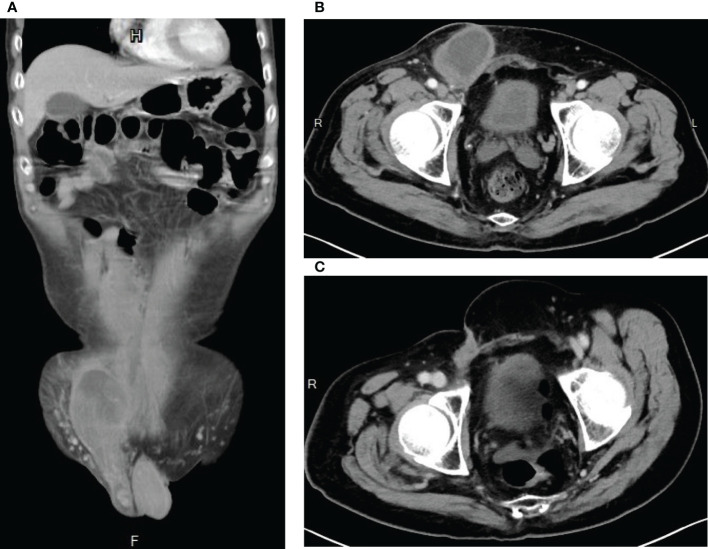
Computed Tomography images showing evidence of tumor shrinkage (A) after neoadjuvant radiotherapy plus conversion surgery, (B) after adjuvant chemotherapy AIM (ifosfamide, Mesna, and doxorubicin) and MAID (Mesna, doxorubincin, ifosfamide, and dacarbazine) regimens, and (C) after ifosfamide chemotherapy.
